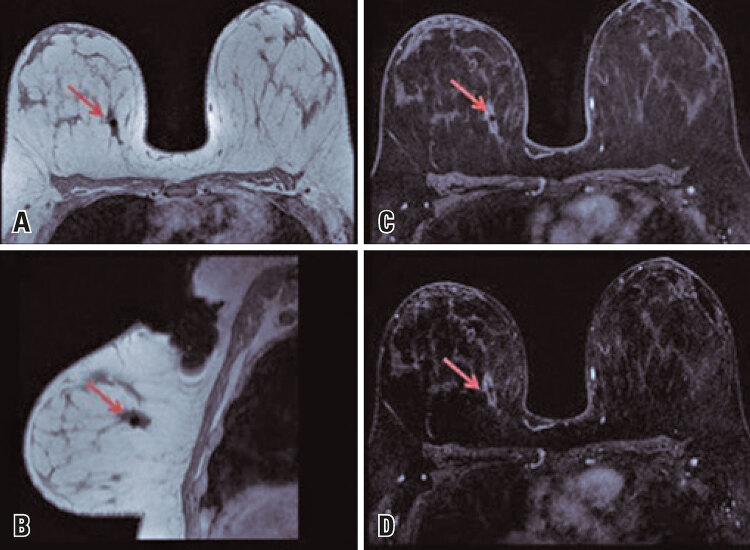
Female, 34 years old, with a palpable mass in the right breast. Biopsy was positive for malignant lesion. Follow-up magnetic resonance imaging after neoadjuvant chemotherapy revealed a post vacuum-assisted biopsy clip contained within an irregular and spiculated mass with peripheral heterogeneous enhancement and measuring 2.5 x 1.2 x 1.1cm. This mass was located in the posterior third of the upper inner quadrant of the right breast (red arrows) and suggested partial response to chemotherapy (classified as BI-RADS 6). (A) Pre-contrast T1 image without fat saturation; (B) Pre-contrast T1 sagittal reconstruction; (C) Second post-contrast T1 sequence; (D) Subtraction image (post-contrast - pre-contrast)
